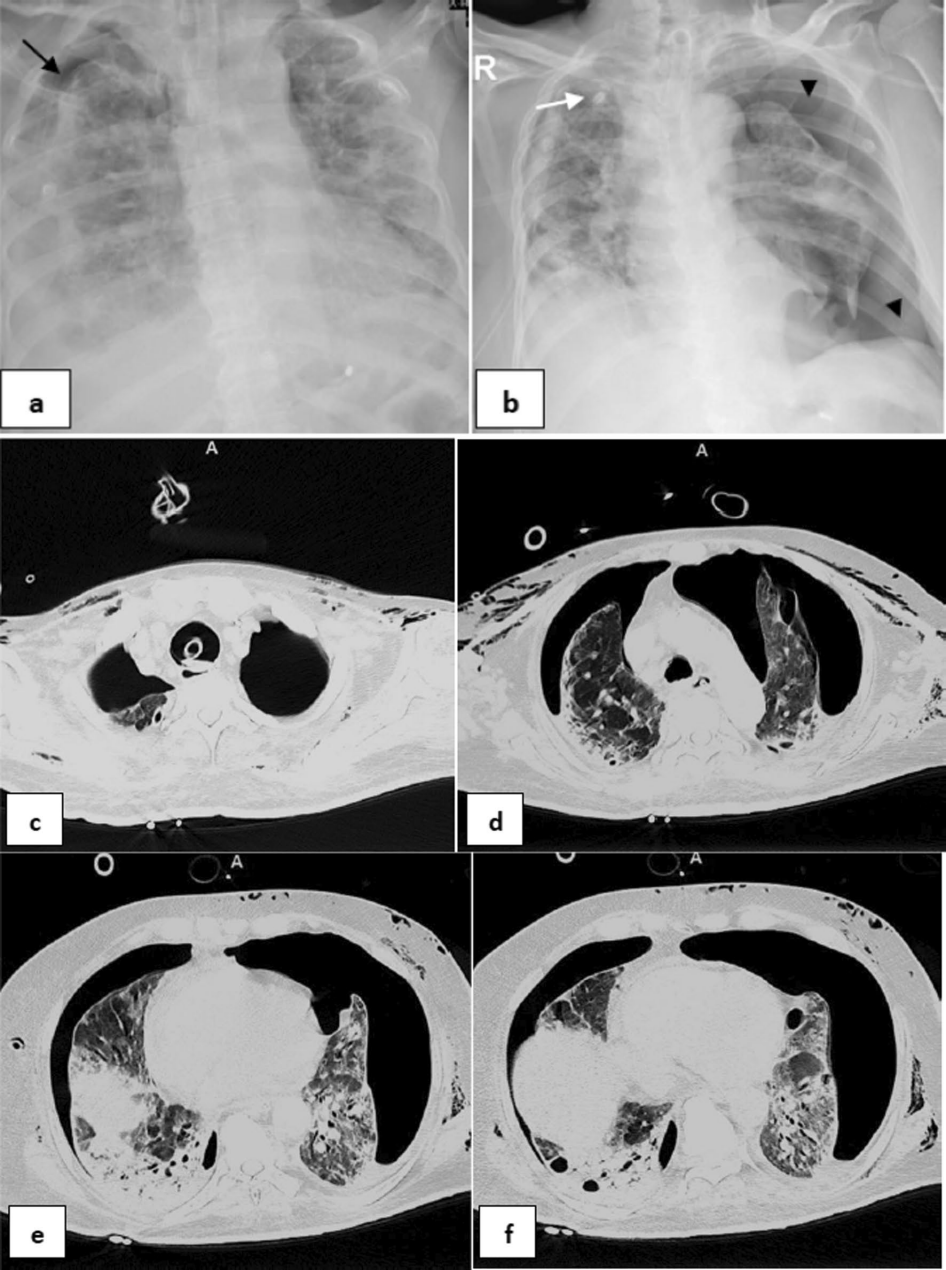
64-year-old female, intubated 5 days post admission. a, b series frontal chest X-ray radiographs demonstrates a small right pneumothorax 9 days after intubation (black arrow), there is a right pleural pigtail catheter (white arrow) followed by marked left pneumothorax (black arrow heads) 1 day later.Note diffuse bilateral ill-defined air space densities throughout both lungs. c–f series axial CT confirming bilateral pneumothoraces
